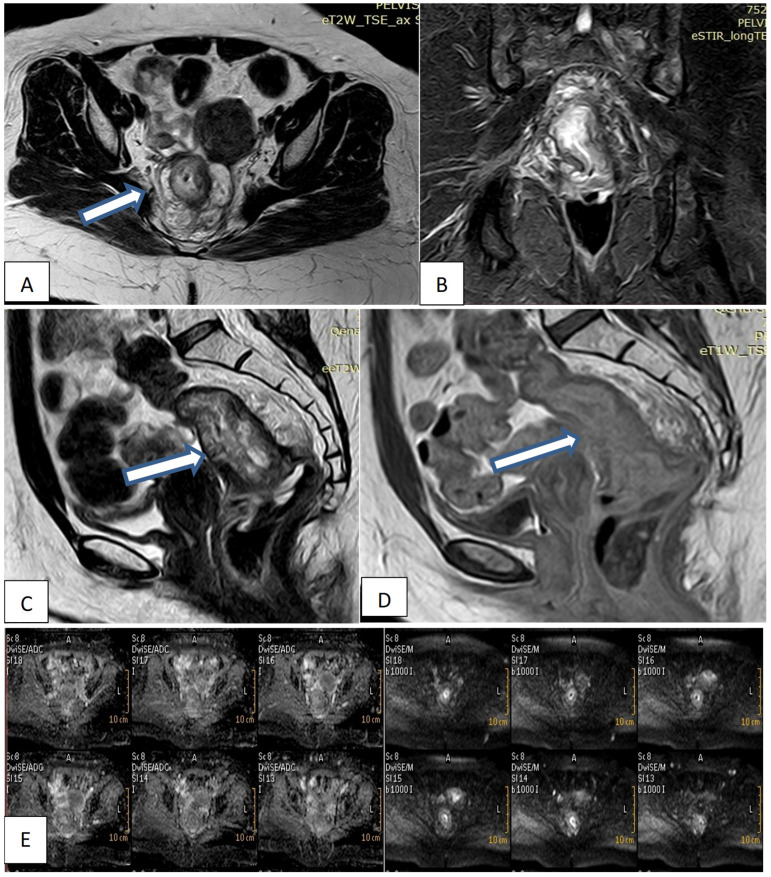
Female patient 47years old with mucinous rectal mass. (A, B, C) Axial & sagittal T2W & coronal STIR MRI images show transmural circumferential thickening about 7.5cm length & 6cm from the anal verge, invading muscularis propria & reaching perirectal fat, not infiltrating levator ani (T3c). (D) Sagittal T1W1 with contrast shows faint enhancement. (E) DWI & ADC images show restricted tumor tissue.
